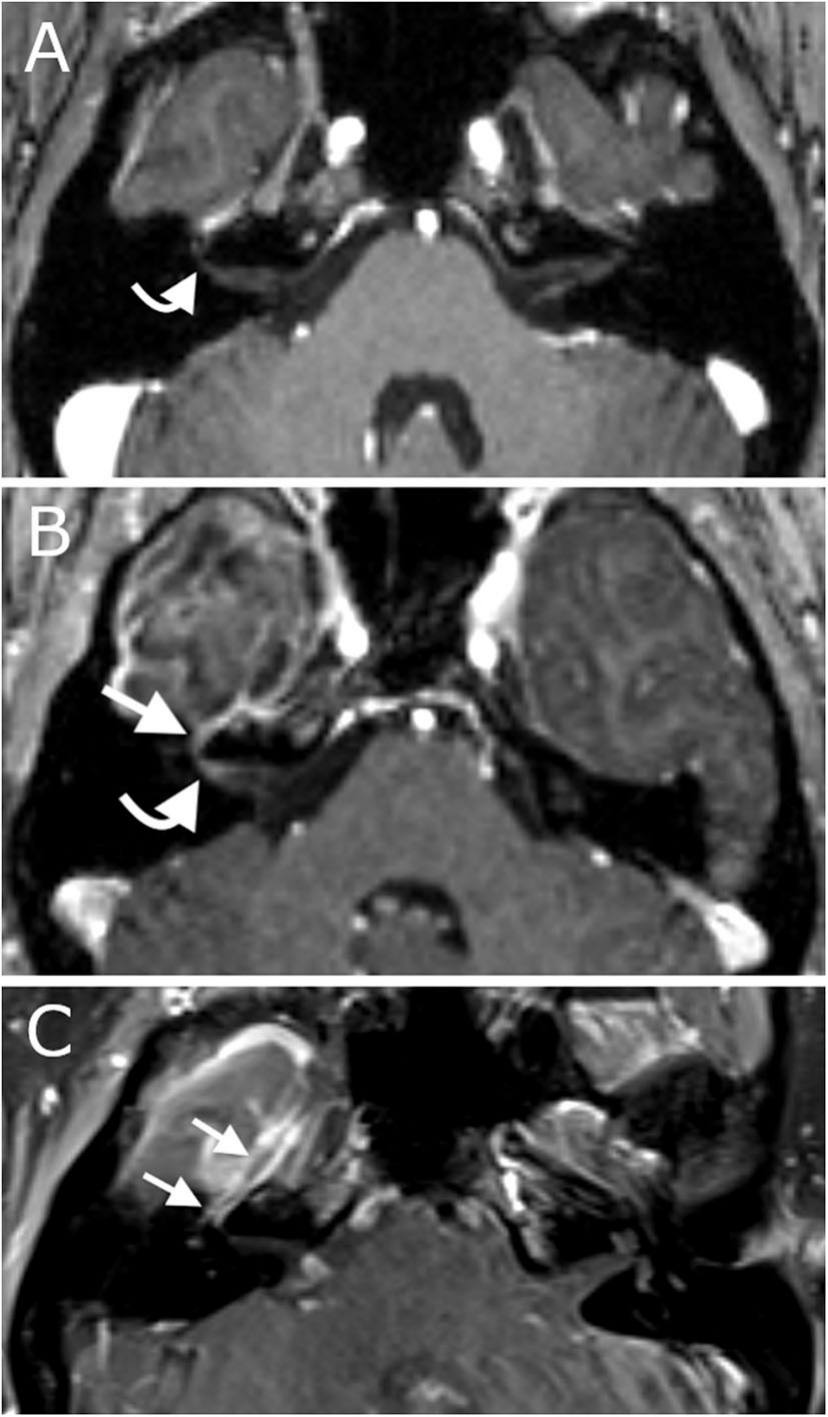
Panel demonstrates MRI scans of the internal acoustic meatus and facial nerve ipsilateral to the ablation. (A) Axial contrast-enhanced T1 pre-operative MRI demonstrates normal appearance of the facial nerve without abnormal enhancement. (B) Axial contrast-enhanced T1 intra-operative MRI demonstrates mild enhancement of the distal canalicular, labyrinthine and geniculate segments of the facial nerve at the time of surgery. (C) Coronal contrast-enhanced T1 MRI demonstrates intense enhancement of the facial nerve 3-months after surgery.
